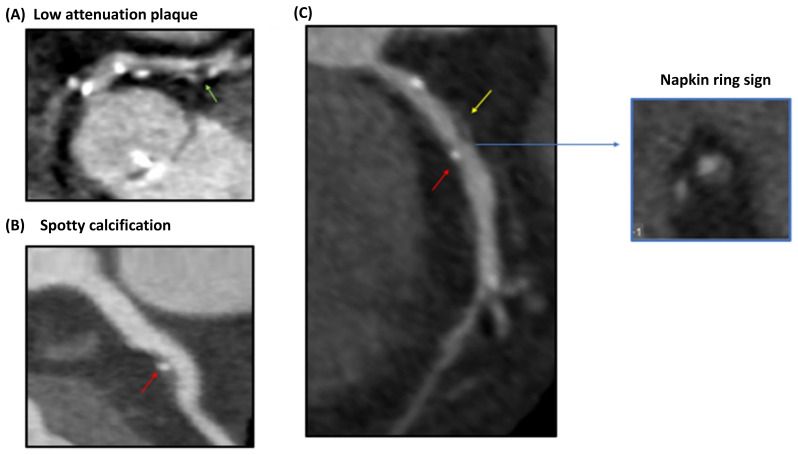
Coronary CT angiography findings of high-risk coronary atherosclerotic plaques. Certain identifiable patterns are indicative of a vulnerable plaque phenotype, namely (A) low plaque attenuation, (B) spotty calcification, and (C) the napkin ring sign. Reproduced with permission from Daghem, M. et al. [59], British Journal of Pharmacology; published by John Wiley & Sons Ltd., 2021, used under Creative Commons CC BY 4.0 license. HU: Hounsfield units. Yellow arrow indicates positive remodeling, red arrow indicates spotty calcification, blue arrow indicates the napkin ring sign.
